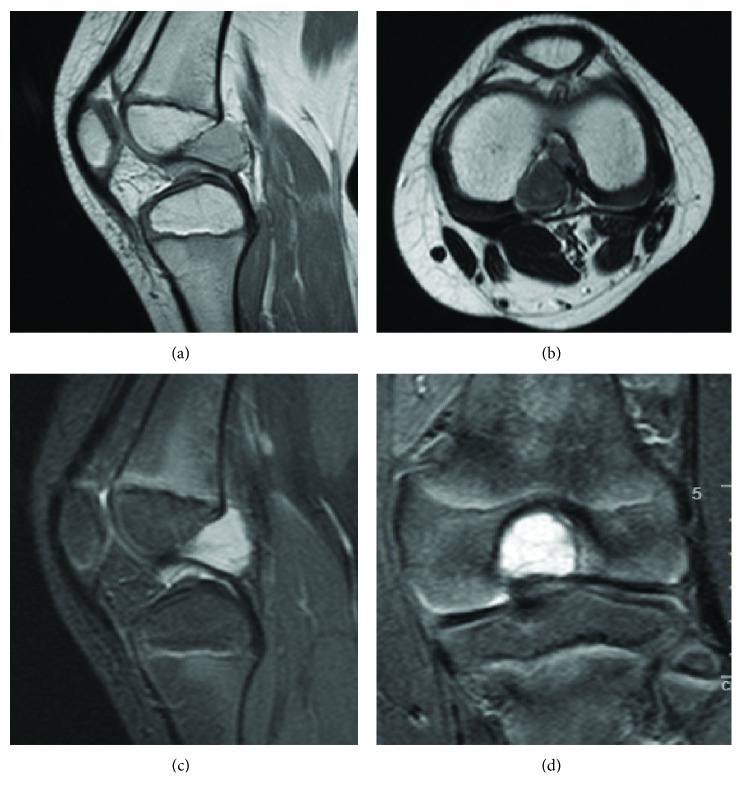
Preoperative magnetic resonance images of the left knee revealed large round-shaped cystic mass encasing the PCL with homogeneous low-signal intensity on sagittal proton density-weighted image (PDWI) (a) and on turbo spin echo (TSE) imaging (b). The lesion appears highly hyperintense on sagittal and coronal turbo inversion recovery magnitude (TIRM) images (c, d).
